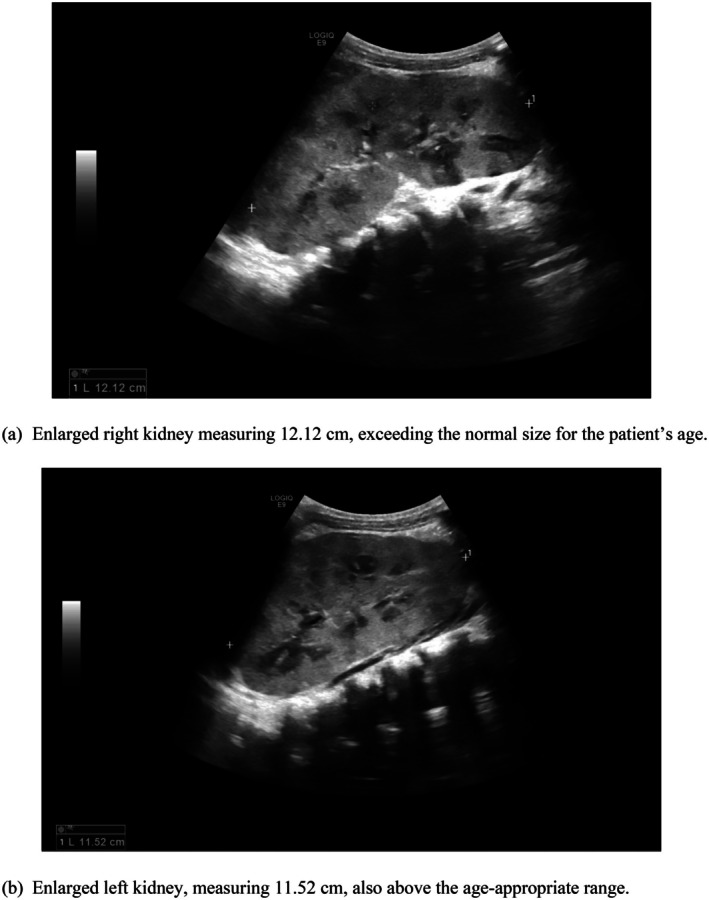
(a, b) Ultrasound of the kidneys upon admission. (a) Enlarged right kidney measuring 12.12 cm, exceeding the normal size for the patient's age. (b) Enlarged left kidney, measuring 11.52 cm, also above the age‐appropriate range.
